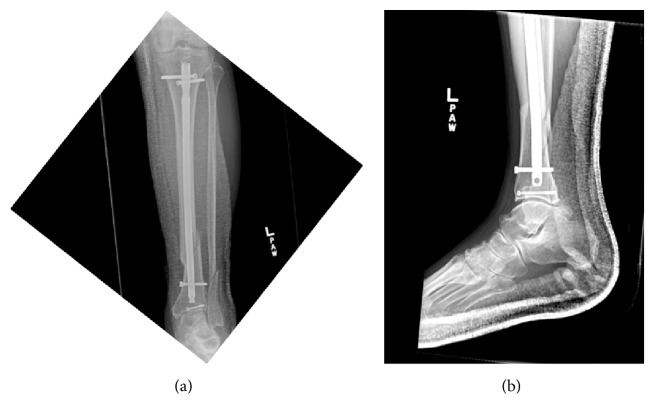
Postoperative radiographs at 4-week follow-up.
